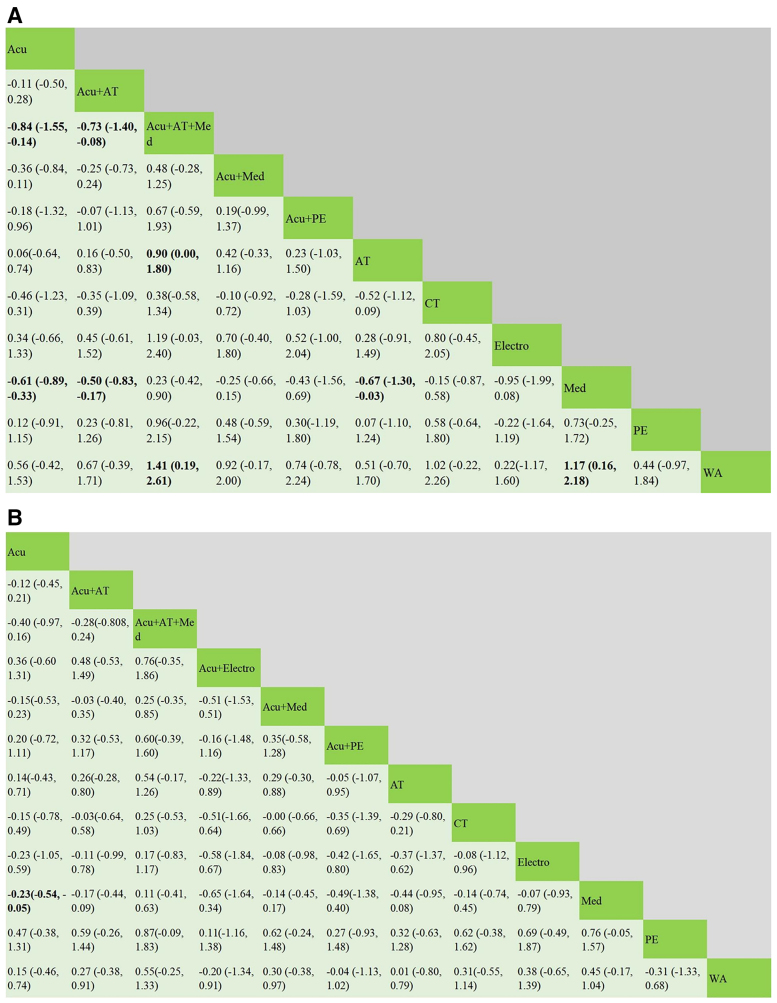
Network meta-analysis results for daytime dysfunction and sleep disturbances. (A) Daytime dysfunction and (B) sleep disturbances. Acu = acupuncture, AT = auriculotherapy, CT = conventional treatment, Electro = electroacupuncture, Med = western medicine, PE = point embedding, WA = warm acupuncture.
